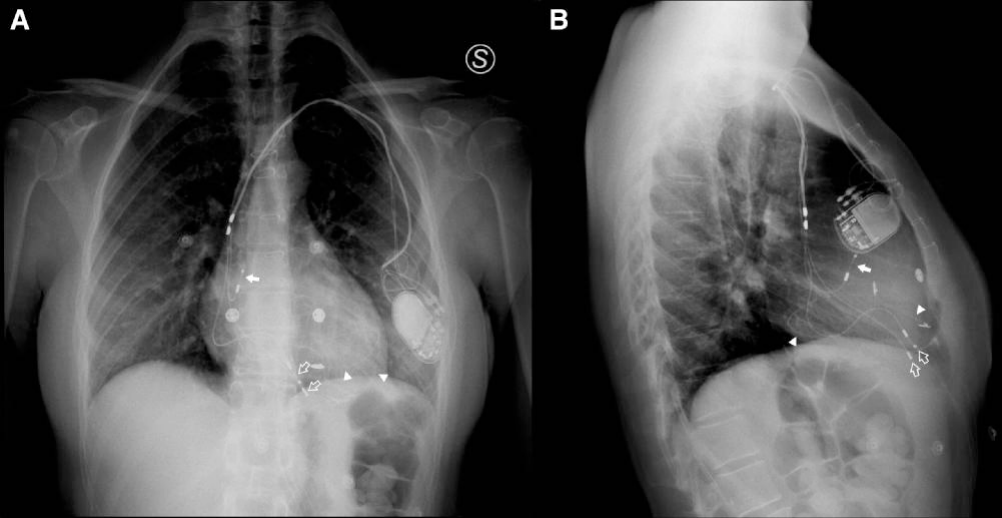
Posterior–anterior (*A*) and lateral (*B*) chest X-rays showing a dual chamber pacemaker generator located in the left antero-lateral chest wall, with the transvenous lead tips located into the right atrial appendage (full arrows) and right ventricular apex (empty arrows), respectively. Multiple abandoned epicardial pacemaker leads along the cardiac surface is also detectable (arrowheads).
